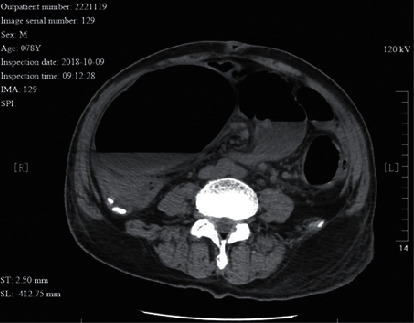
A 78 year-old male patient with megacolon complicated with volvulus. The CT image shows a whirl sign. Bowel wall ischemia and mesenteric venous thrombosis were observed during surgery.
